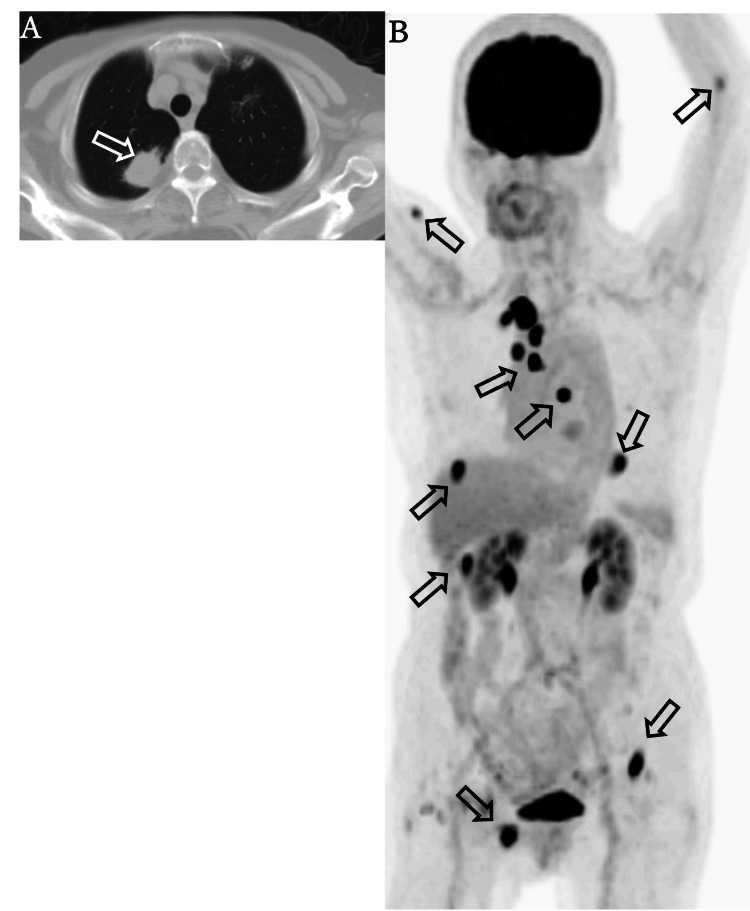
Chest CT (pulmonary window) and PET-CT scan. (A) A nodular shadow with pleural indentation is observed in the S1 segment of the right upper lung lobe, within the lung field striae. (B) Increased maximum standardized uptake value (SUVmax) accumulation is noted in the hilar lymph nodes (#3a, 4R), liver S8 region, left proximal radius, right pubis, myocardium, right kidney, right brachialis, left inguinal iliac region, and right 3rd-4th intercostal spaces, all considered metastatic lesions from lung cancer.
